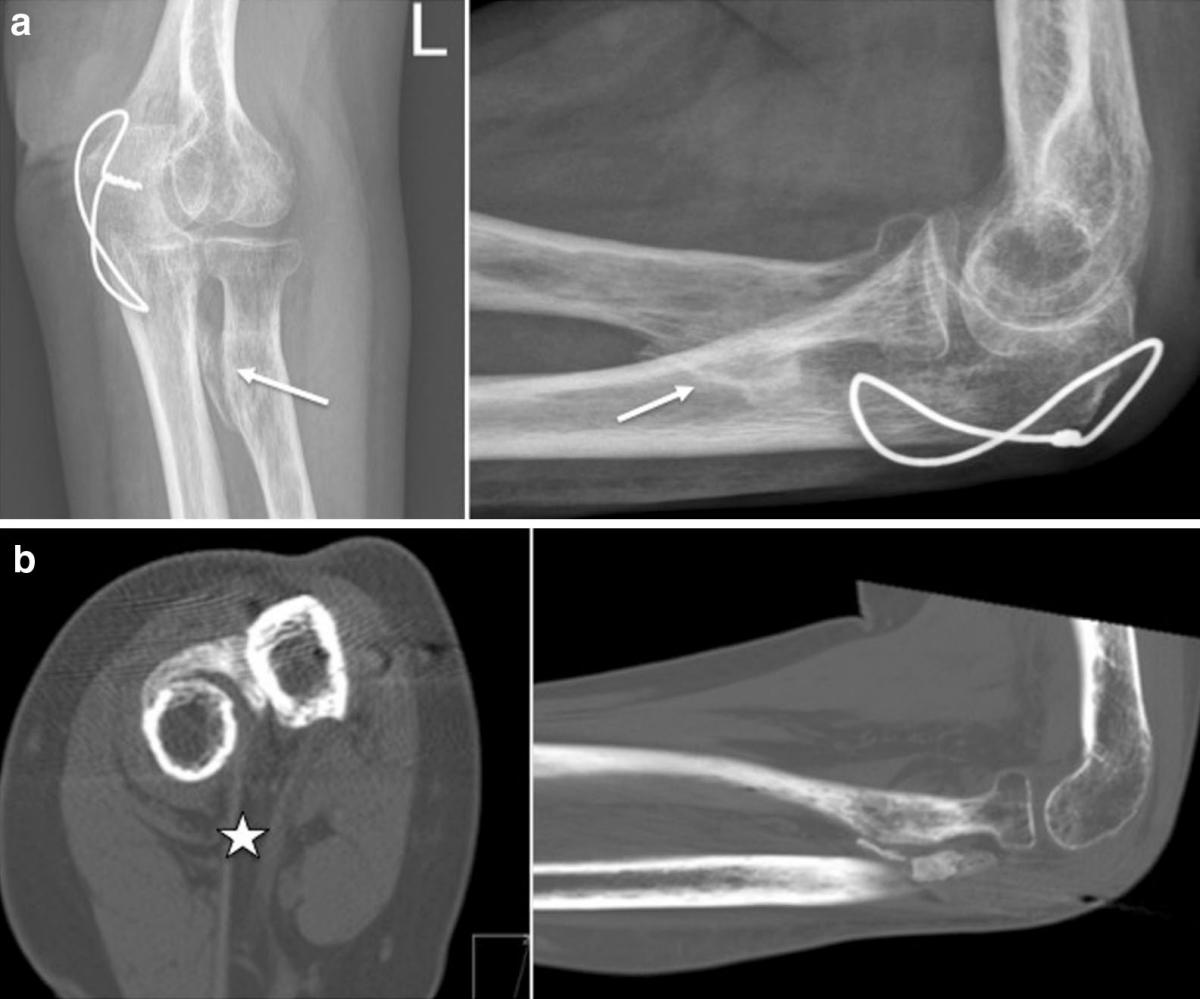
Proximal radioulnar synostosis. a Massive radioulnar synostosis (*white arrows*) at the level of the radial tuberosity 3 months after initial surgery. The olecranon fracture is healed while the cerclage wire remained in situ. b CT scans show the heterotopic ossification starting from the distal biceps tendon (*white star*) insertion to the ulnar cortex
